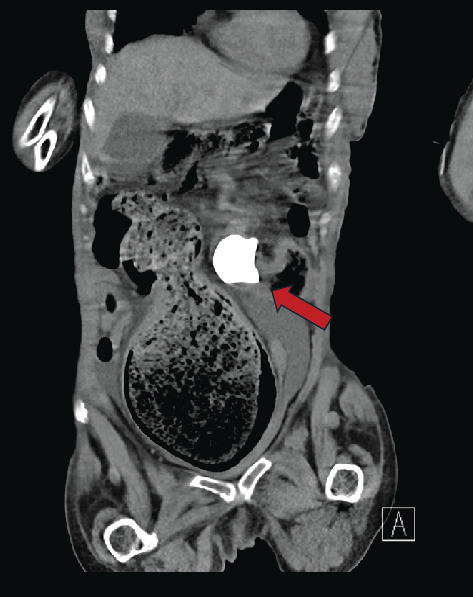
Coronal plane of the initial CT scan, showing the staghorn calculus within the renocolic fistula, measuring 4.5 cm. The migrated staghorn is seen adjacent to the distended large bowel and not in the anatomical position of the right renal pelvis.
